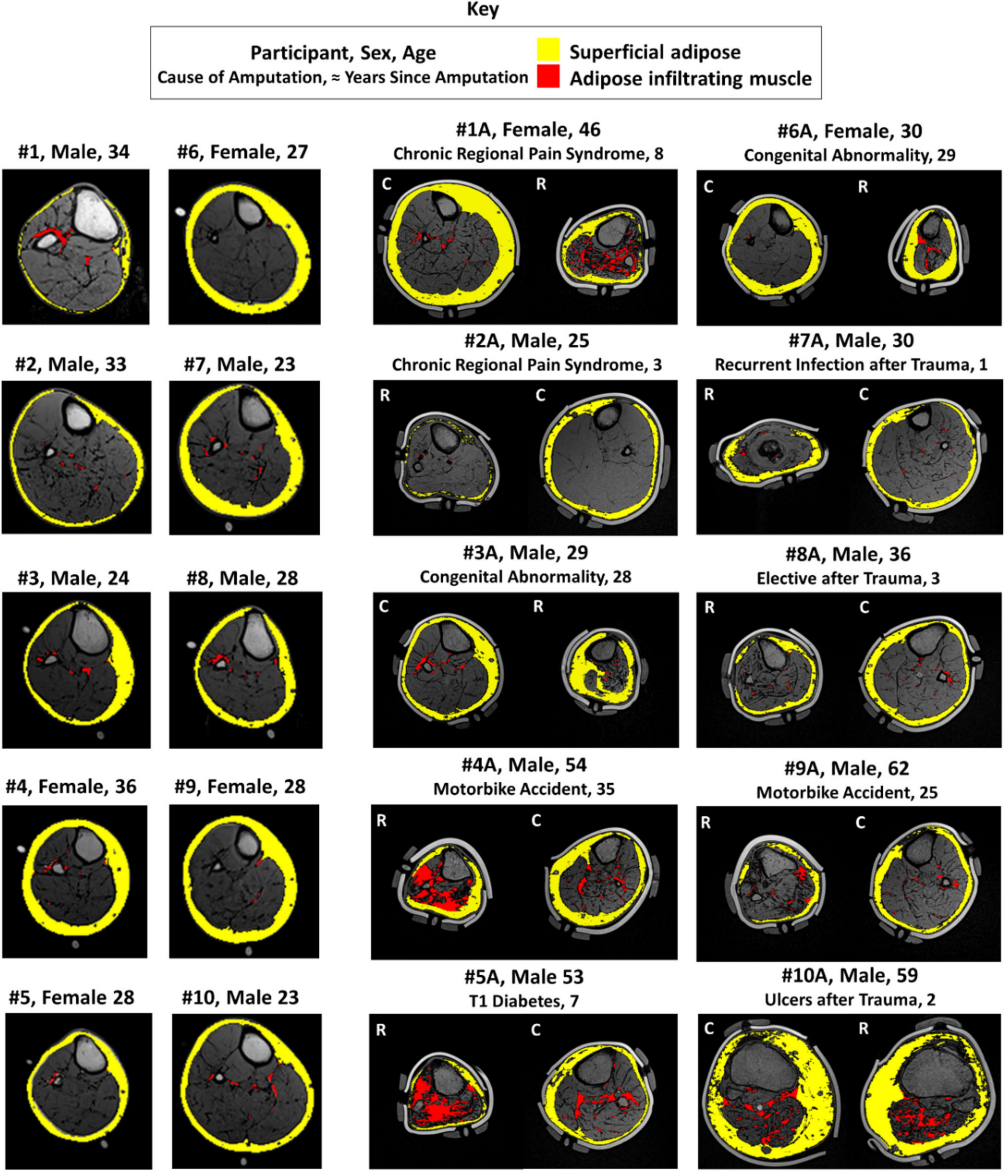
Exemplar transverse MRI slices in the calf with superficial adipose (yellow) and adipose infiltrating muscle (red) tissue overlays. Images represent the right control limb of ten participants without amputation (left columns #1-10), and both the control (C) and residual (R) limbs for ten participants with transtibial amputation (right columns, #1A-#10A).
